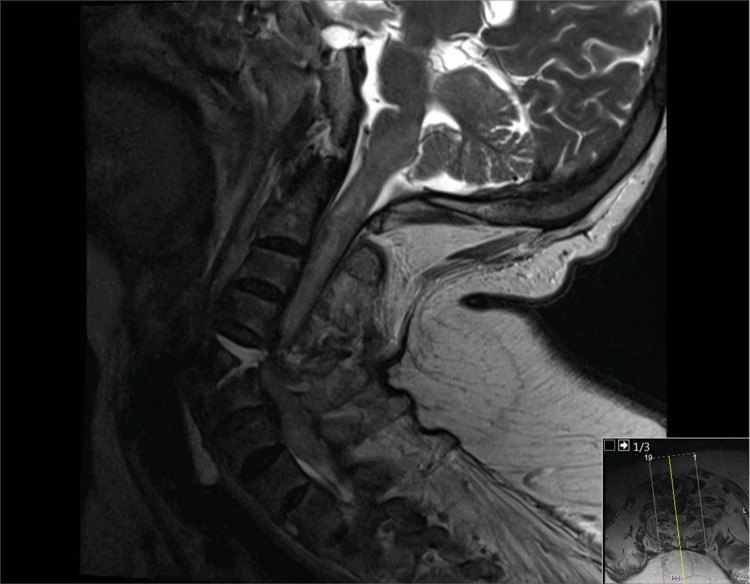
Cervical spine MRI in the sagittal T2-weighted sequence revealing a disruption injury at the C4-5 disk site and spinal cord compression. In addition, there is extensive spinal cord edema extending to the brain stem
